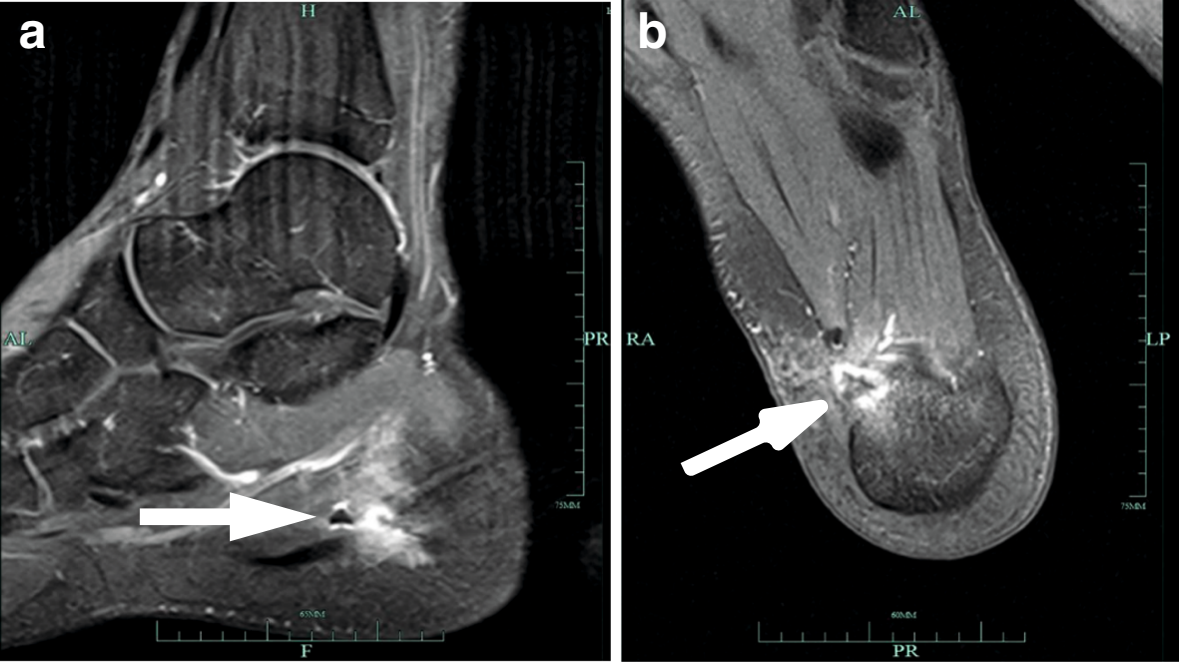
T1-weighted MRI. a Sagittal view. b Horizontal view. Figures show a minor calcaneal spur, with severe signs of inflammation at the calcaneal tubercle (see *arrows*) surrounded by a large edema, and structural changes of the plantar fascia
